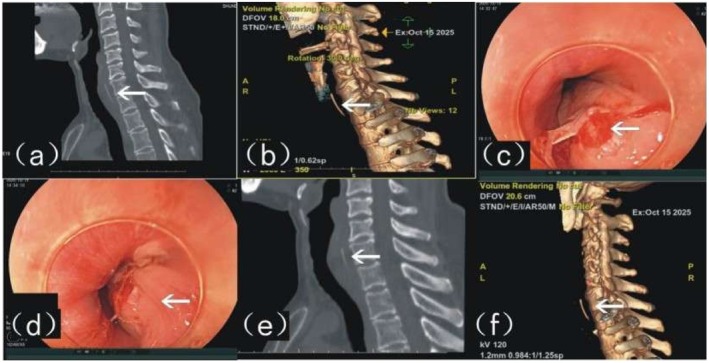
(a) The CT scan revealed a fish bone like foreign body in the upper esophagus (white arrow). (b) Three‐dimensional CT reconstruction confirmed the foreign body anterior to the sixth and seventh cervical vertebrae (white arrow). (c) The Upper GI endoscopy revealed swelling at the entrance of the esophagus, with the friable mucosa and white secretions. (d) Submucosal tumor‐like swelling of the esophagus. (e) Repeat CT confirming persistence of the foreign body (white arrow). (f) The repeat CT three‐dimensional reconstruction showing unchanged location and size of the foreign body.
